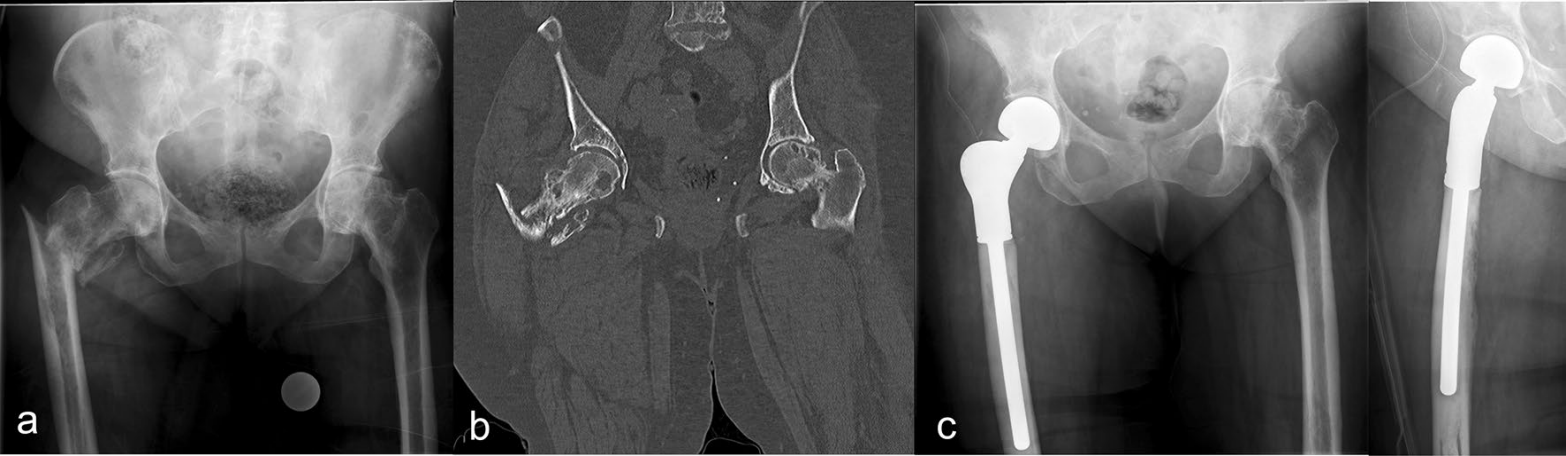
A pathological femoral fracture due to metastatic breast cancer, shown on preoperatively x-ray (a) and CT-scan (b), that was treated with a proximal femoral replacement (c).
